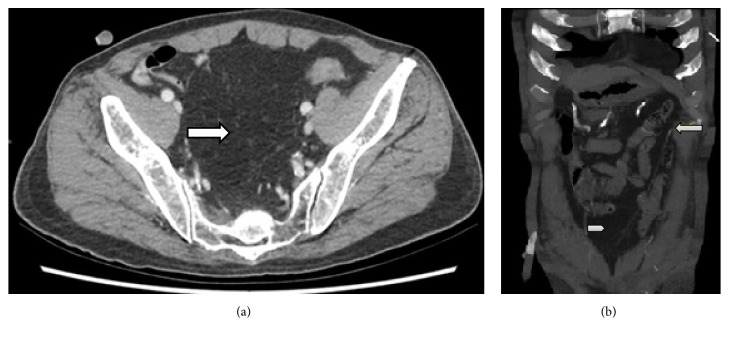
Case 3: Figures 4(a) and 4(b). (a) Axial CT image in the arterial phase showing a large fat-containing mass in the pelvis displacing bowel loops. No contrast extravasation is seen. (b) Coronal MIP CT images in the arterial phase showing aberrant vessel arising from the splenic artery (white arrow) and coursing obliquely from the left hypochondrium to the region of the pelvic mass (white arrowhead).
